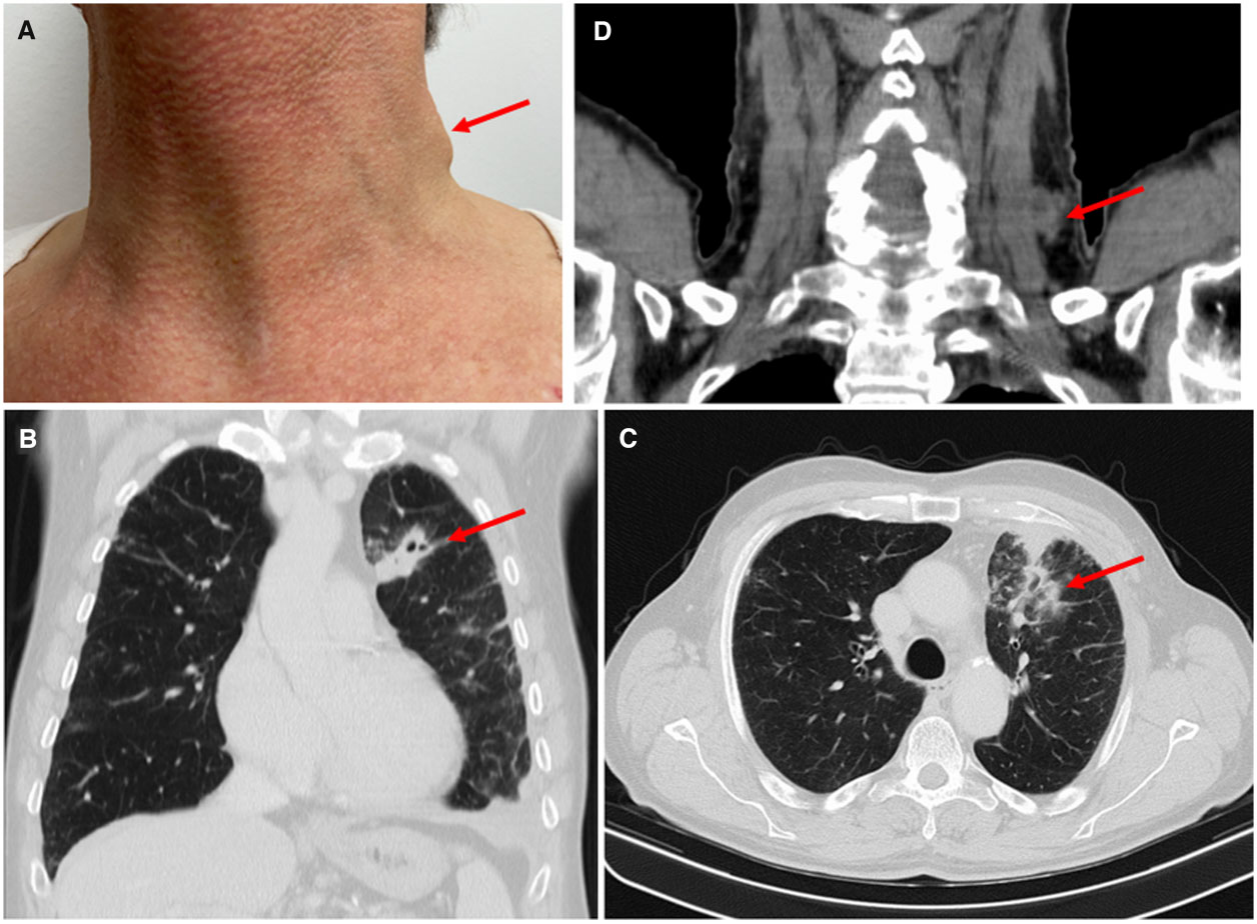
Lymph node and pulmonary tuberculosis during upadacitinib treatment (A) Tumour in the left side of the neck. (B and C) Consolidation in the left lung in CT scan. (D) Nodule in the left side of the neck in CT scan.
